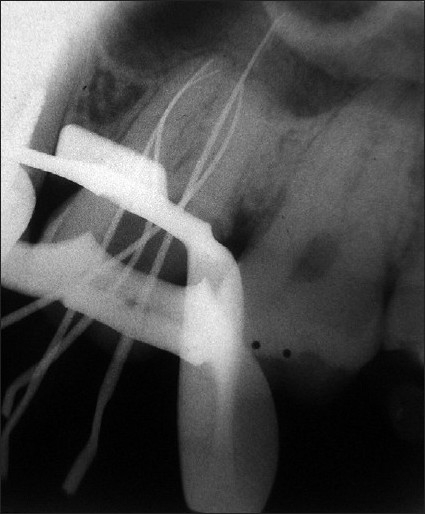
Intra oral radiograph showing working length in relation to # 14
